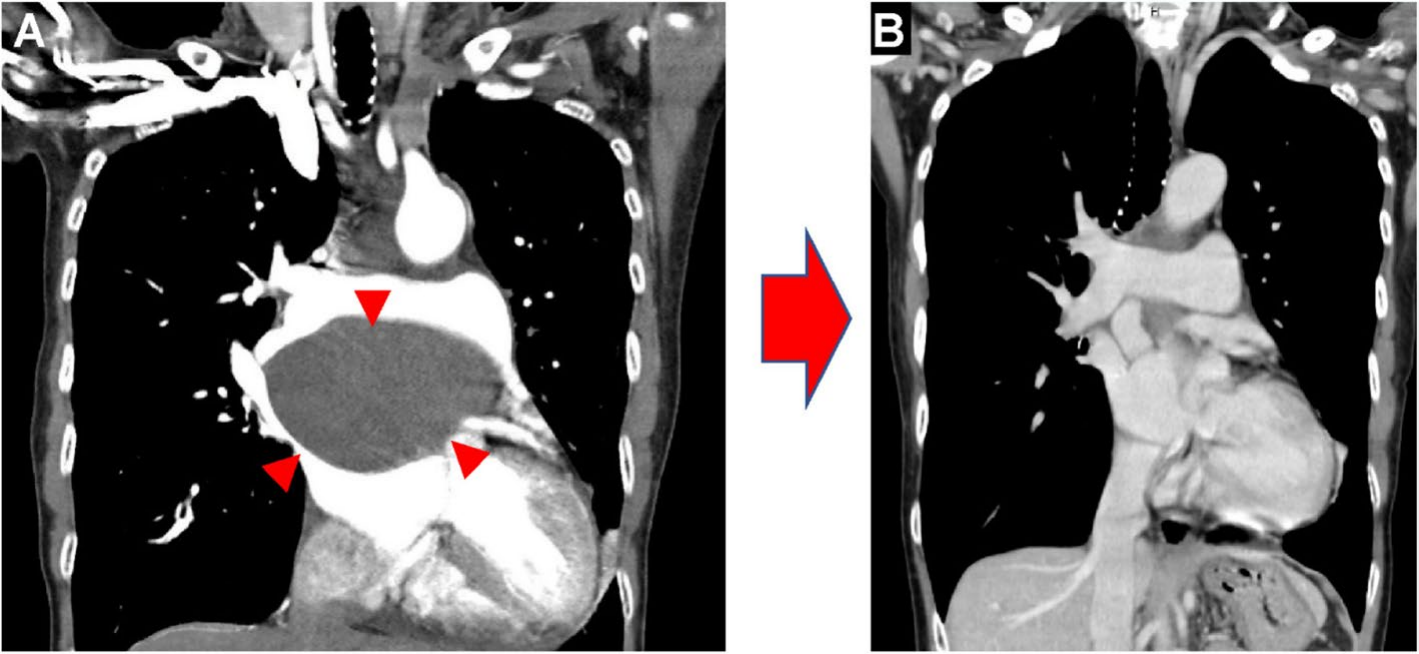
Chest CT images. A The cystic lesion (arrowhead) is encountered preoperatively. B The cystic lesion is completely resected postoperatively
